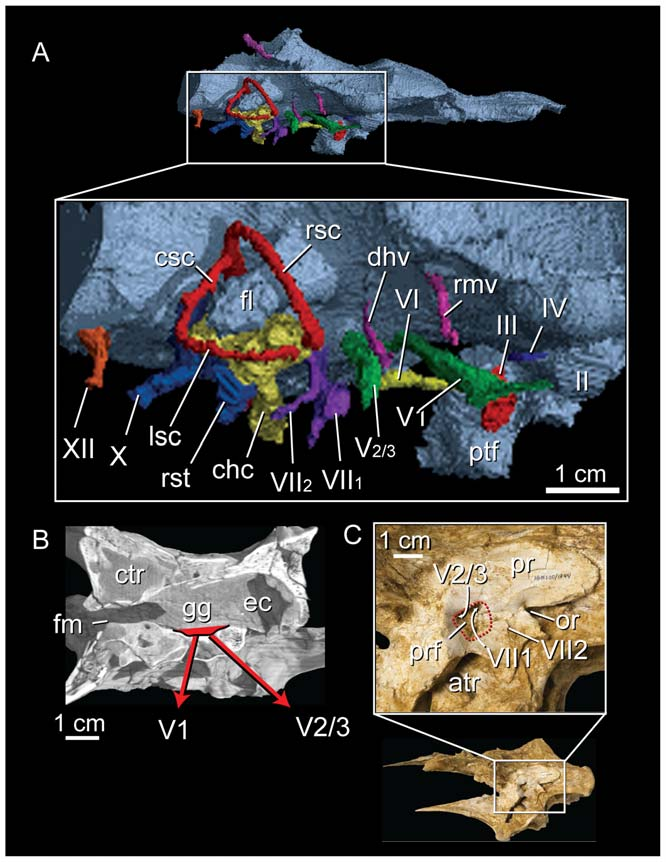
Morphology of the cranial nerve roots in *Alioramus altai* (IGM 100/1844). (A) Digital reconstruction of the cranial nerve roots in right dorsolateral view. (B) Horizontal CT slice showing the position of the gasserian ganglion and the split paths of the ophthalmic and maxillomandibular branches of the trigeminal nerve through the braincase. C) External view of the left lateral surface of the braincase showing the position of the prootic fossa, which accepts the lateral opening of the maxillomandibular branch of the trigeminal nerve and the palatine branch of the facial nerve. II, optic canal; III, oculomotor canal; IV, trochlear canal; V_1_, ophthalmic ramus of the trigeminal canal; V_2/3_, maxillomandibular ramus of the trigeminal canal; VI, abducens canal; VII_1_, palatine ramus of facial canal; VII_2_, hypoglossal ramus of facial canal; X, vagal canal; XII, hypoglossal canal; atr, anterior tympanic recess; chc, cochlear canal; csc, caudal semicircular canal; cts, caudal tympanic sinus; dhv, dorsal head vein canal; fl, floccular canal; fm, foramen magnum; gg, fossa housing gasserian ganglion; lsc, lateral semicircular canal; or, otic recess; ptf, pituitary fossa; rsc, rostral semicircular canal; rmv, rostral middle cerebral vein canal; rst, recessus scalae tympani.
